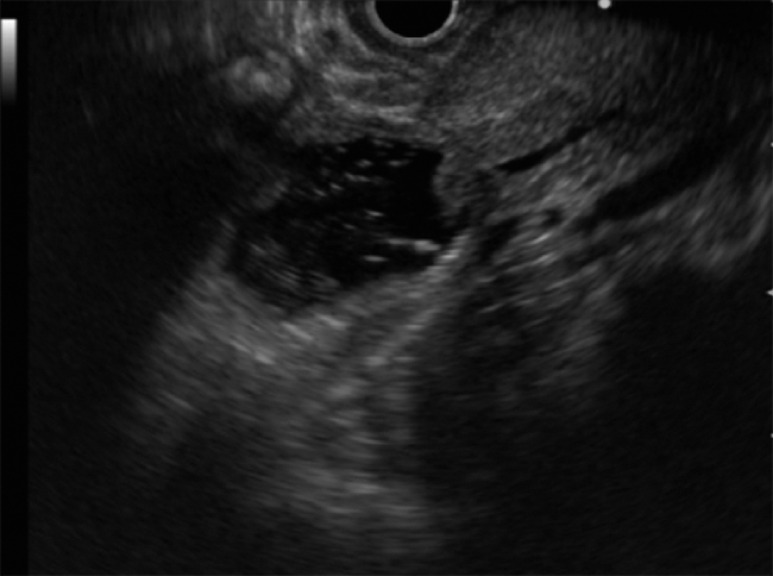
Gel-immersion endoscopic ultrasonography (GI-EUS) of a 43-year-old woman admitted for examination of suspected pancreaticobiliary maljunction. GI-EUS, like the underwater technique, provides excellent visualization of the duodenal ampulla. Here, GI-EUS shows a normal confluence between the pancreatic duct and bile duct, ruling out pancreaticobiliary maljunction.
